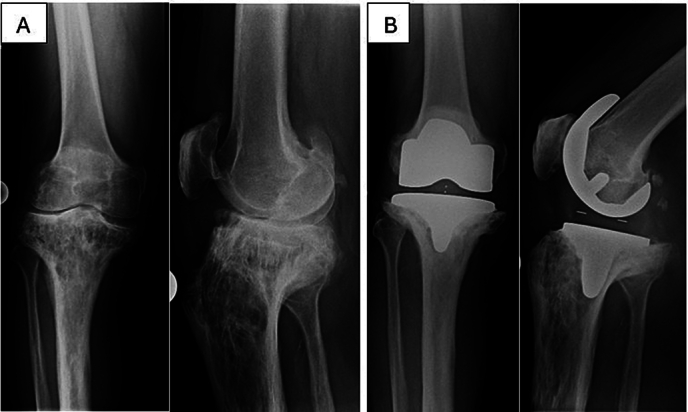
A Anteroposterior (AP) and lateral radiographs of the right knee from 2013 showing Paget's disease of the proximal tibia prior to TKA. B Postoperative AP and lateral radiographs of the right knee following TKA in 2013.
